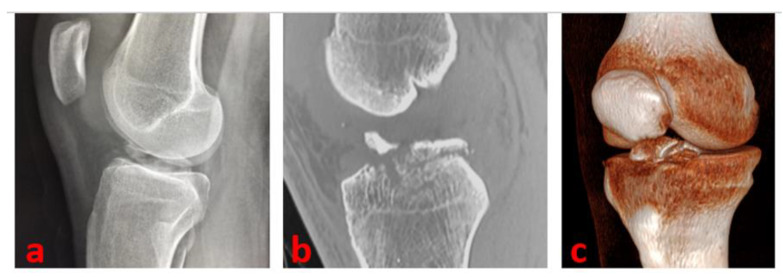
A true lateral knee radiographic view of the right knee of a 16-year-old male patient of our series shows a Type 3 tibial avulsion fracture (a); CT scans are useful to define the pattern of the fracture and drive the management: CT sagittal view scan (b) and 3D CT reconstruction (c) of the same patient.
